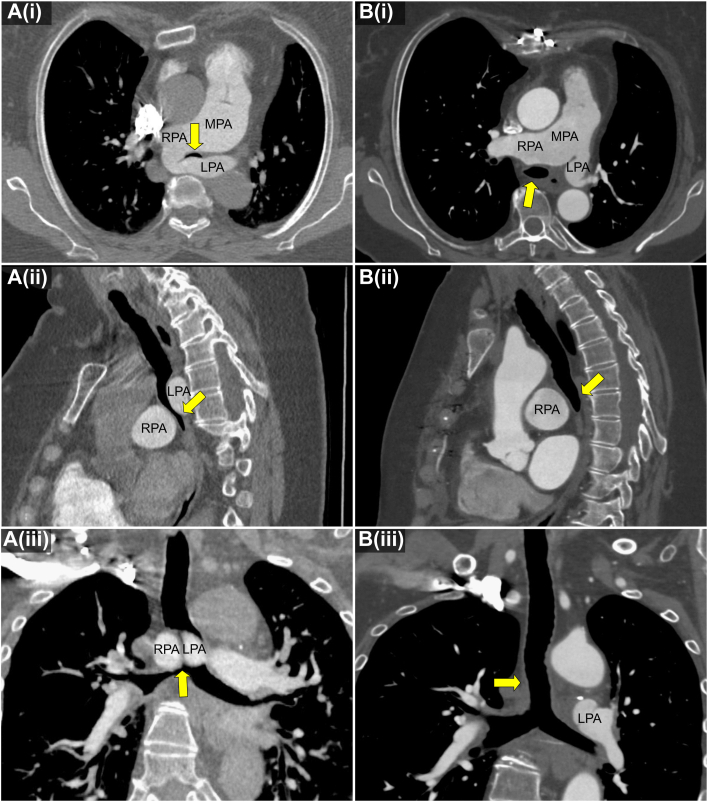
Computed tomography before and after left pulmonary artery sling repair. (A) Preoperative computed tomography shows significant tracheal compression (arrow) between the right and left pulmonary arteries, as visualized in (i) axial, (ii) sagittal, and (iii) coronal planes. (B) Postoperative imaging shows marked improvement in tracheal patency at the site of prior compression (arrow). (LPA, left pulmonary artery; MPA, main pulmonary artery; RPA, right pulmonary artery.)
